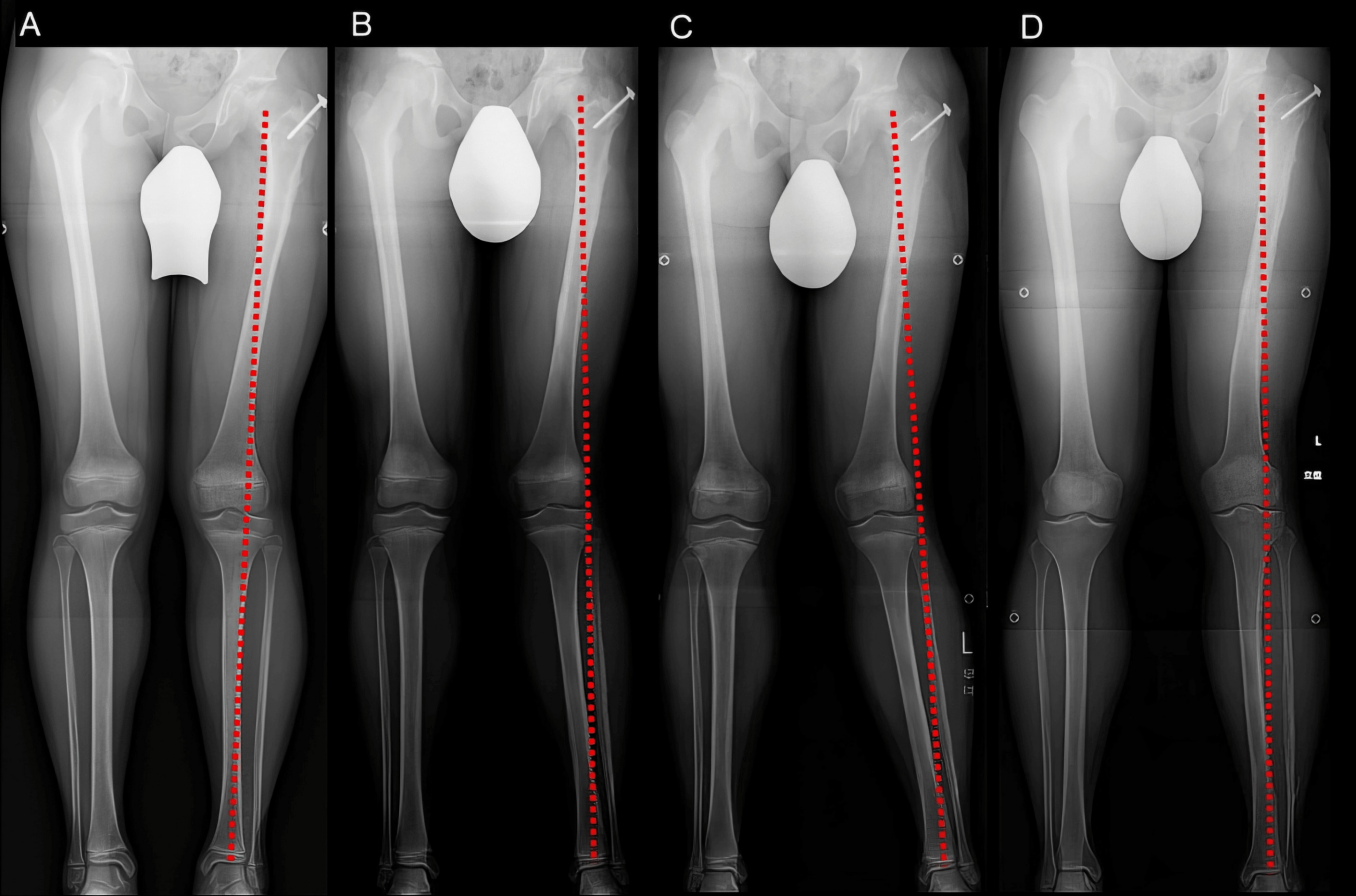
Whole-leg radiographs of a patient who showed a transient genu valgum A: Before PSPD. B: One year after PSPD. C: Two years after PSPD. D: Three years after PSPD PSPD: periosteal stripping and periosteal division
